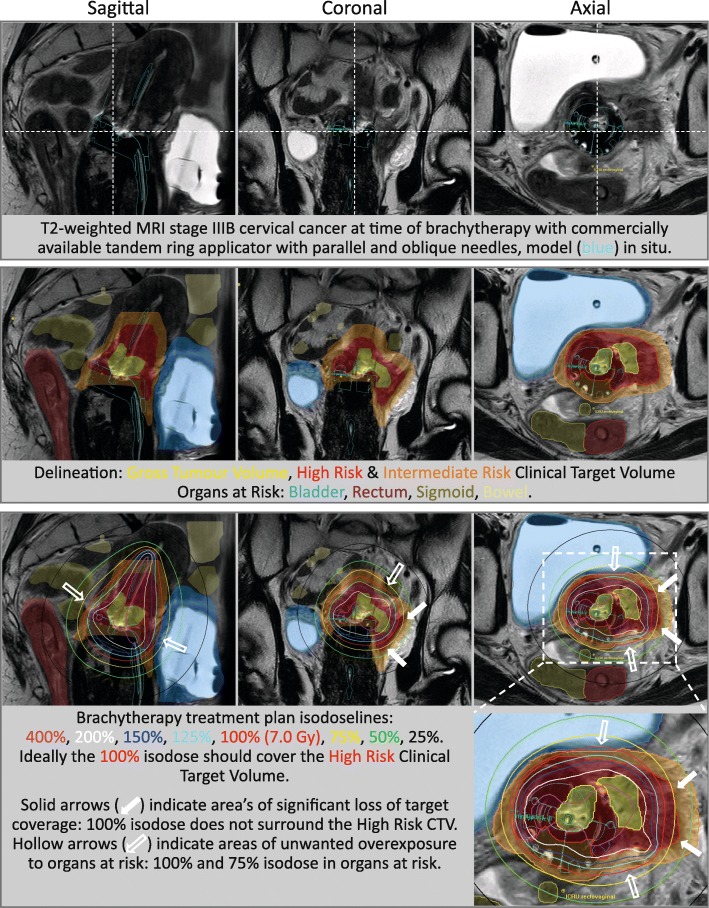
Limitations of an advanced standard applicator with parallel and oblique interstitial needles is demonstrated in a patient with a tumour involving the lateral parametrium. The delineation of relevant anatomy on T2 weighted MRI and the overlay of BT treatment plan isodose lines are shown. Underexposure of target volumes and overexposure of organs at risk are indicated by solid and hollow arrows
